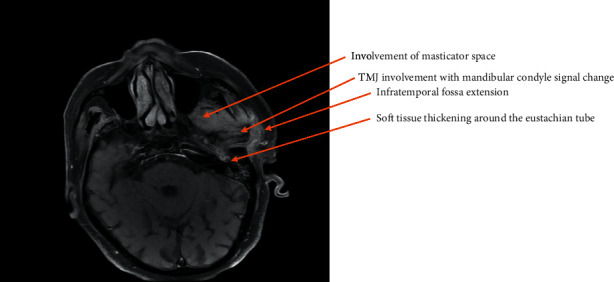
T1-weighted axial section MRI demonstrating left-sided necrotising otitis externa involving the left temporomandibular joint before treatment.
